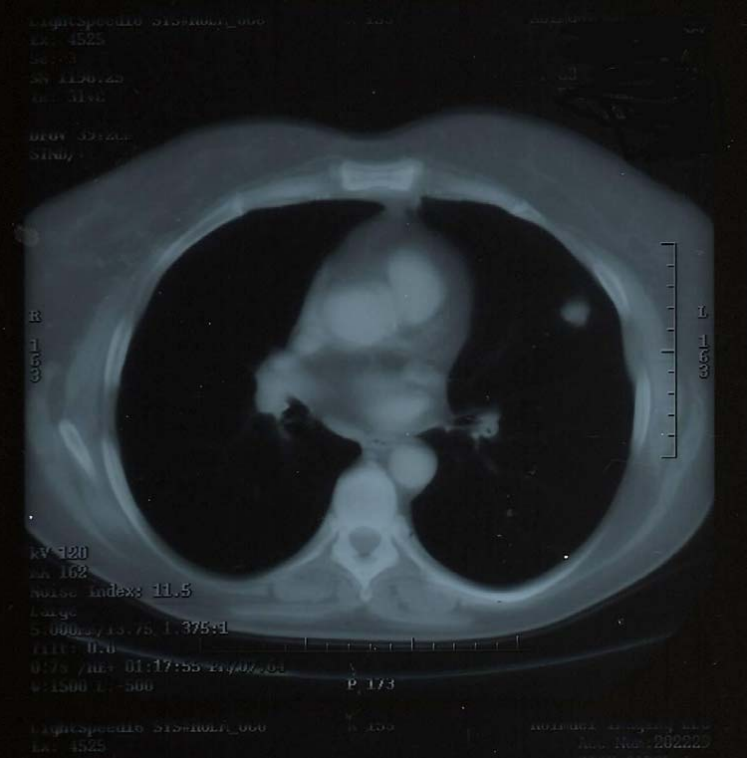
Chest CT scan. Left upper lobe pulmonary nodule.
